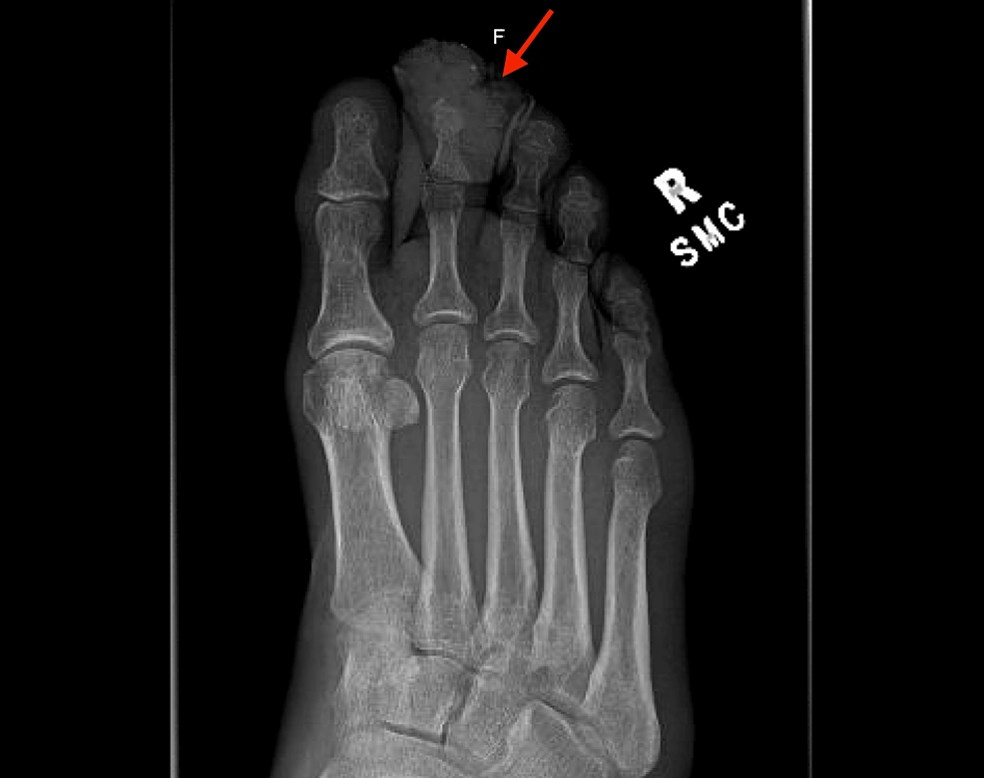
Anteroposterior X-ray of the right foot showing fracture of the second middle phalangeal head and soft tissue swelling.
